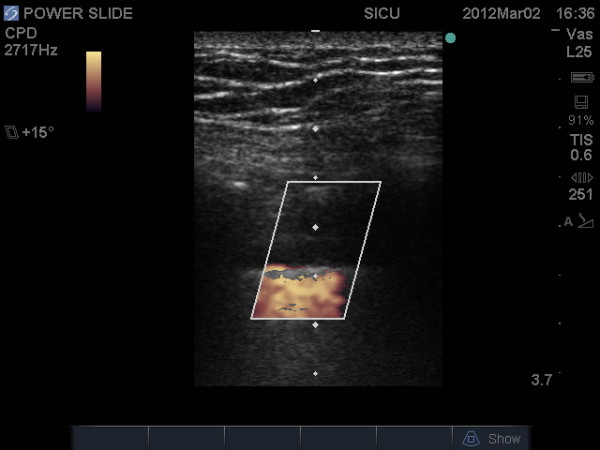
The "power slide" - Power color doppler image indicating motion at the pleural line confirming the presence of lung sliding.
